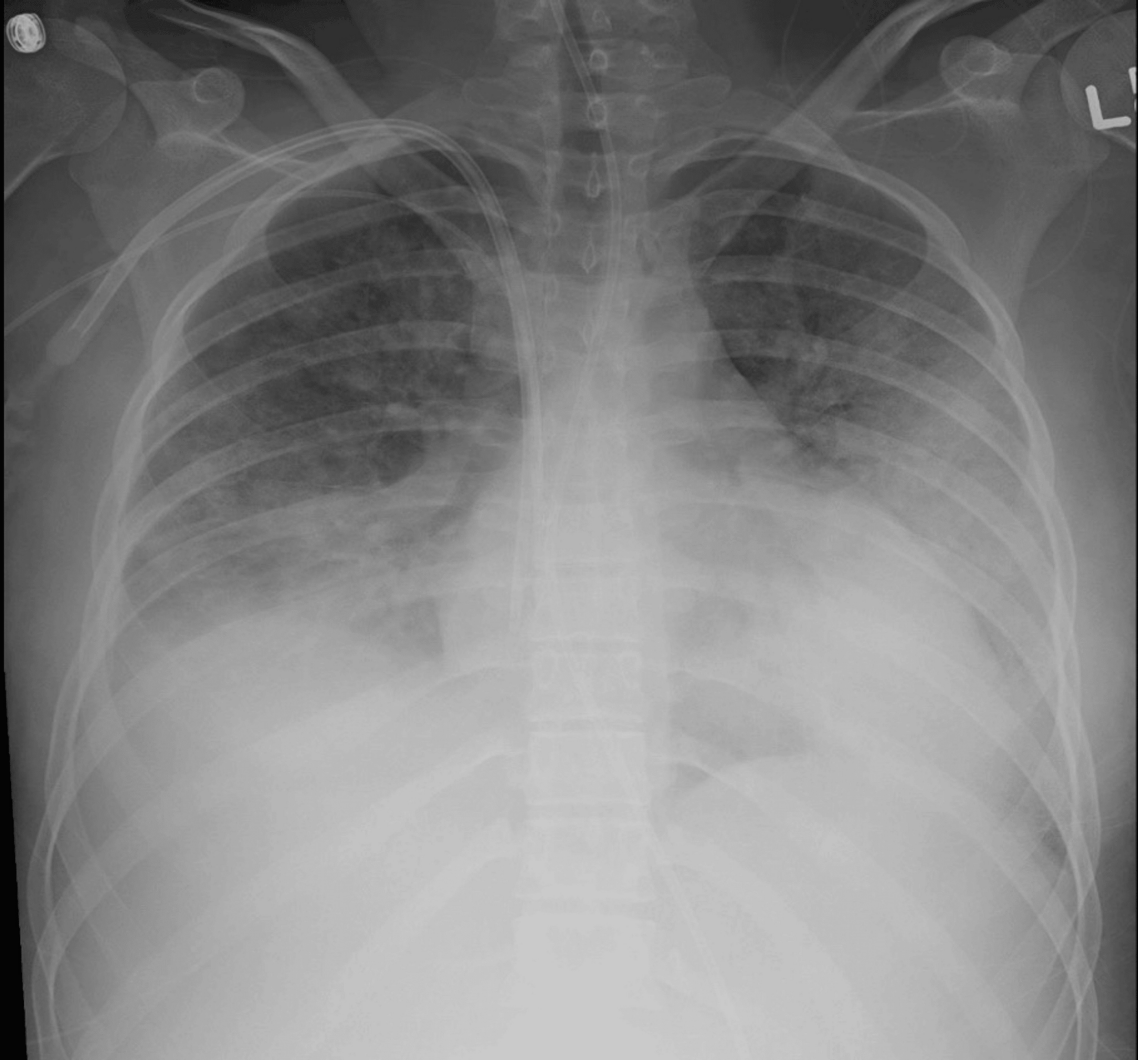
A 16-year-old child with lymphoma and secondary HLH Chest radiograph demonstrates bilateral alveolar and interstitial opacities and right pleural effusion. Right-sided venous access catheters have been placed for treatment. HLH: hemophagocytic lymphohistiocytosis
